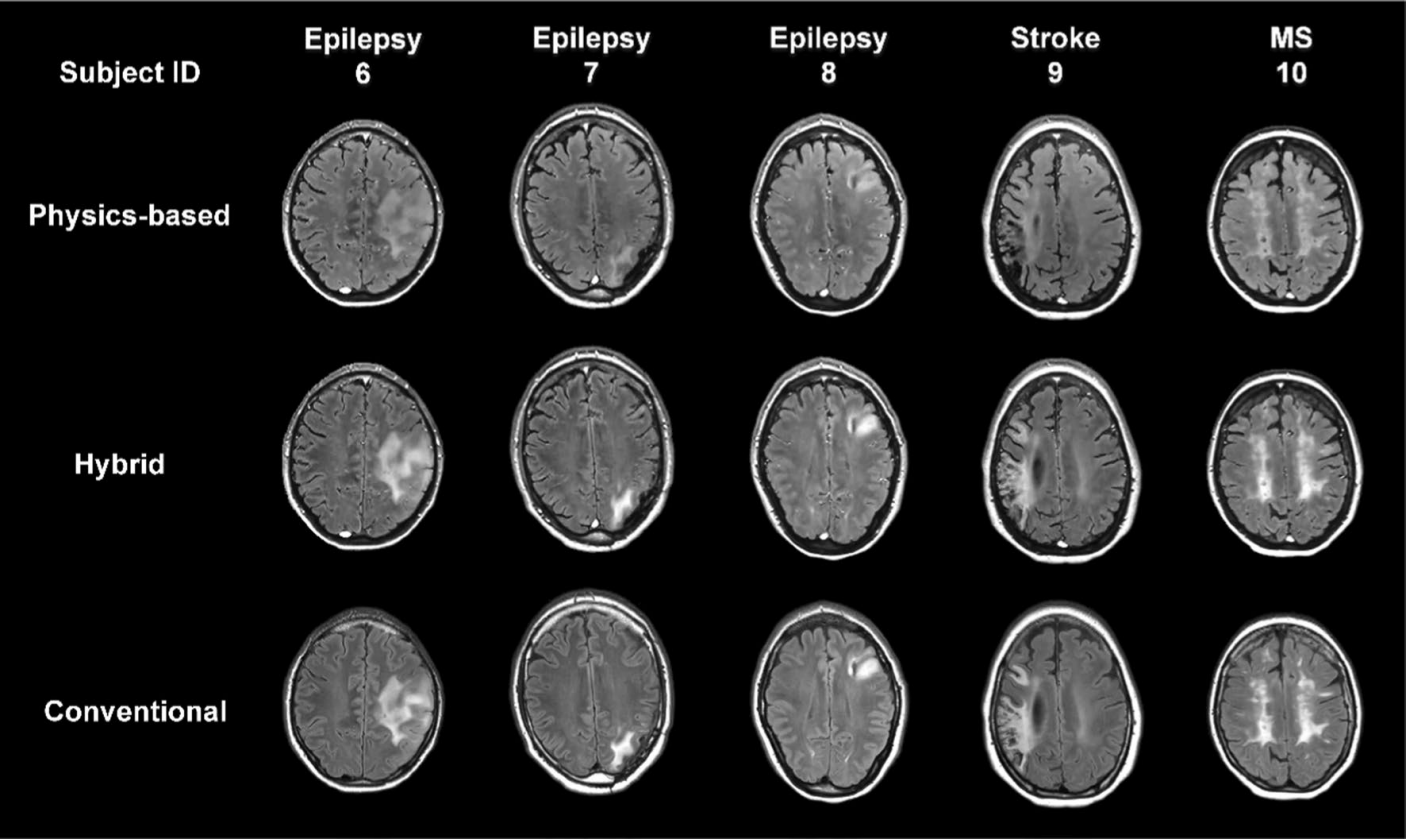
Comparison of FLAIR images as obtained from the physics-based model, the hybrid method and conventional FLAIR. The axial slices of interest from representative patients with (6)-(8) epilepsy, (9) stroke, and (10) multiple sclerosis are shown
